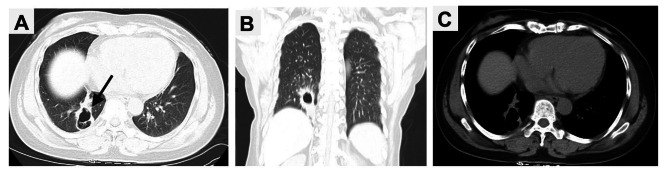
A 42-year-old female patient underwent routine CT examination on January 06, 2022, and found a round-like cavity in the right lung with irregular inner wall, and its size was about 59 mm×31 mm (black arrow). (A) Axial lung window;(B) Coronal lung window; (C) Axial soft tissue window
